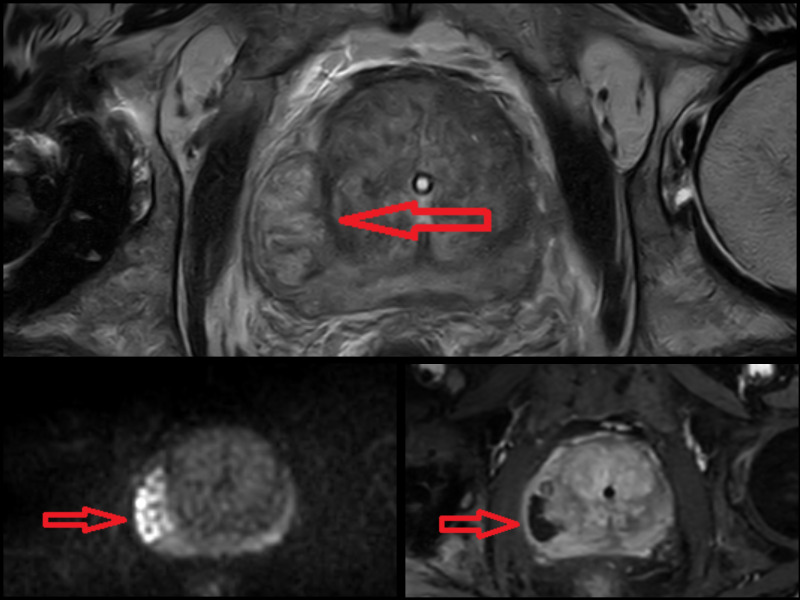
Magnetic resonance imaging of the prostate showing a prostatic abscess on T2-weighted imaging, diffusion-weighted imaging, and contrast-enhanced sequences (arrows).
